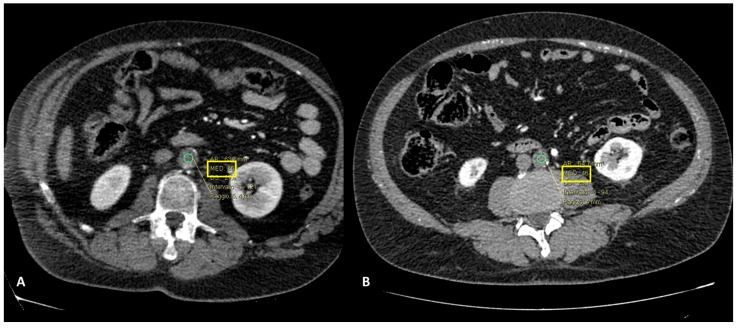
CT angiography, axial planes, arterial phase. In (A), the ROI placed in the aortic thrombus showed a value of 65 HU, while in (B), the value showed was 46 HU. Patient in (A) presented a chronic aortic occlusion while patient in (B) was one of the patients included in our study, affected by primary AAO (male, age 54 y/o).
